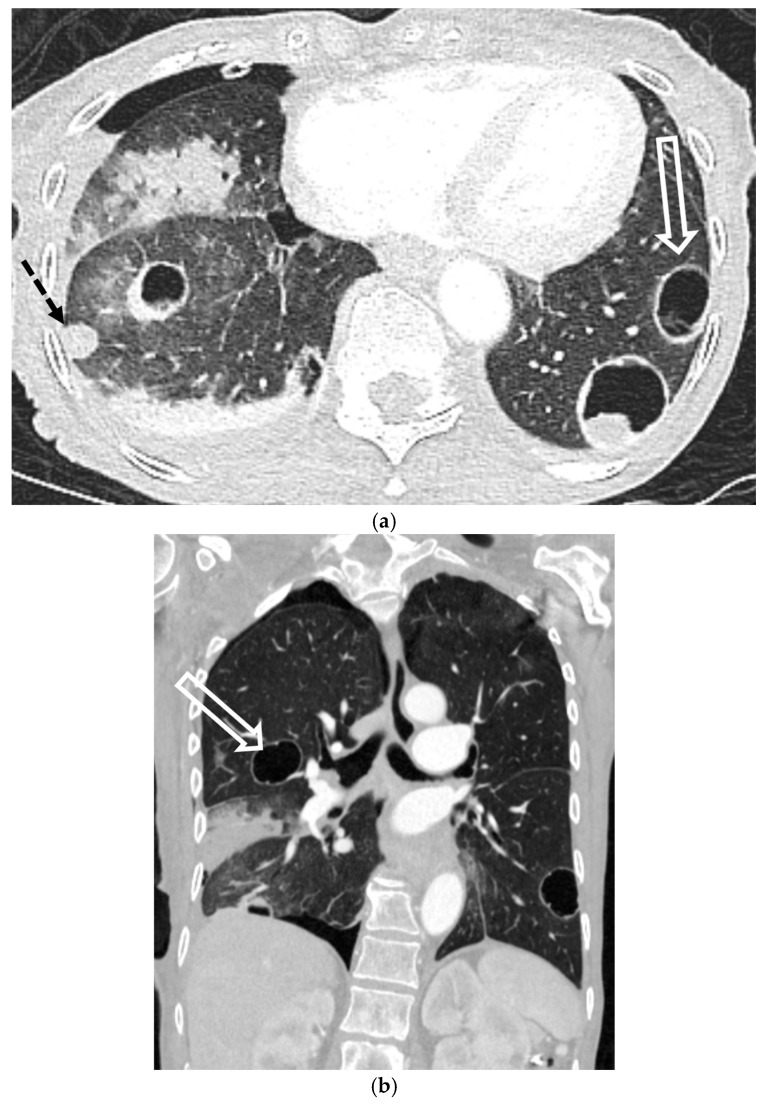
69-year-old female with a history of hepatocellular carcinoma presents with chest pain. Axial and coronal CT images in lung window (a,b) show small right pneumothorax, status post right chest tube placement, as well as multiple thick-walled cavitary lesions (open white arrows) and solid lung nodules.
